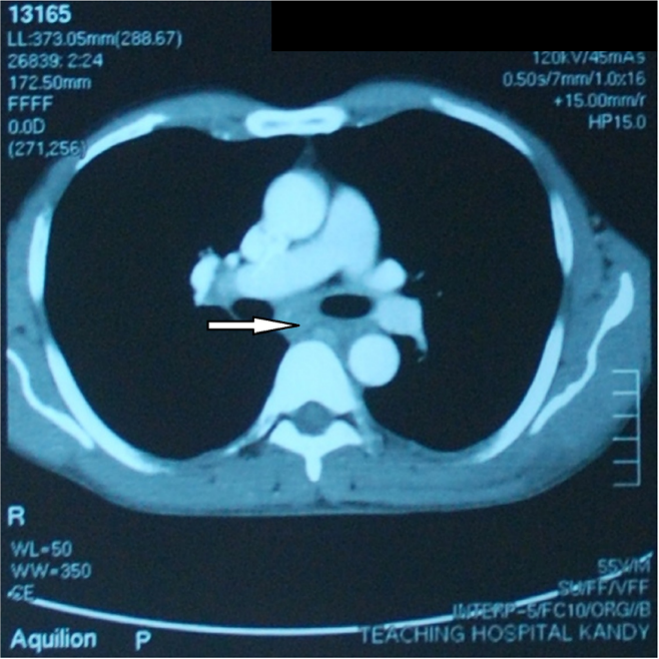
Contrast enhanced computed tomography scan of chest showing sub-carinal lymphadenopathy. (Arrow indicates sub-carinal lymphadenopathy).
